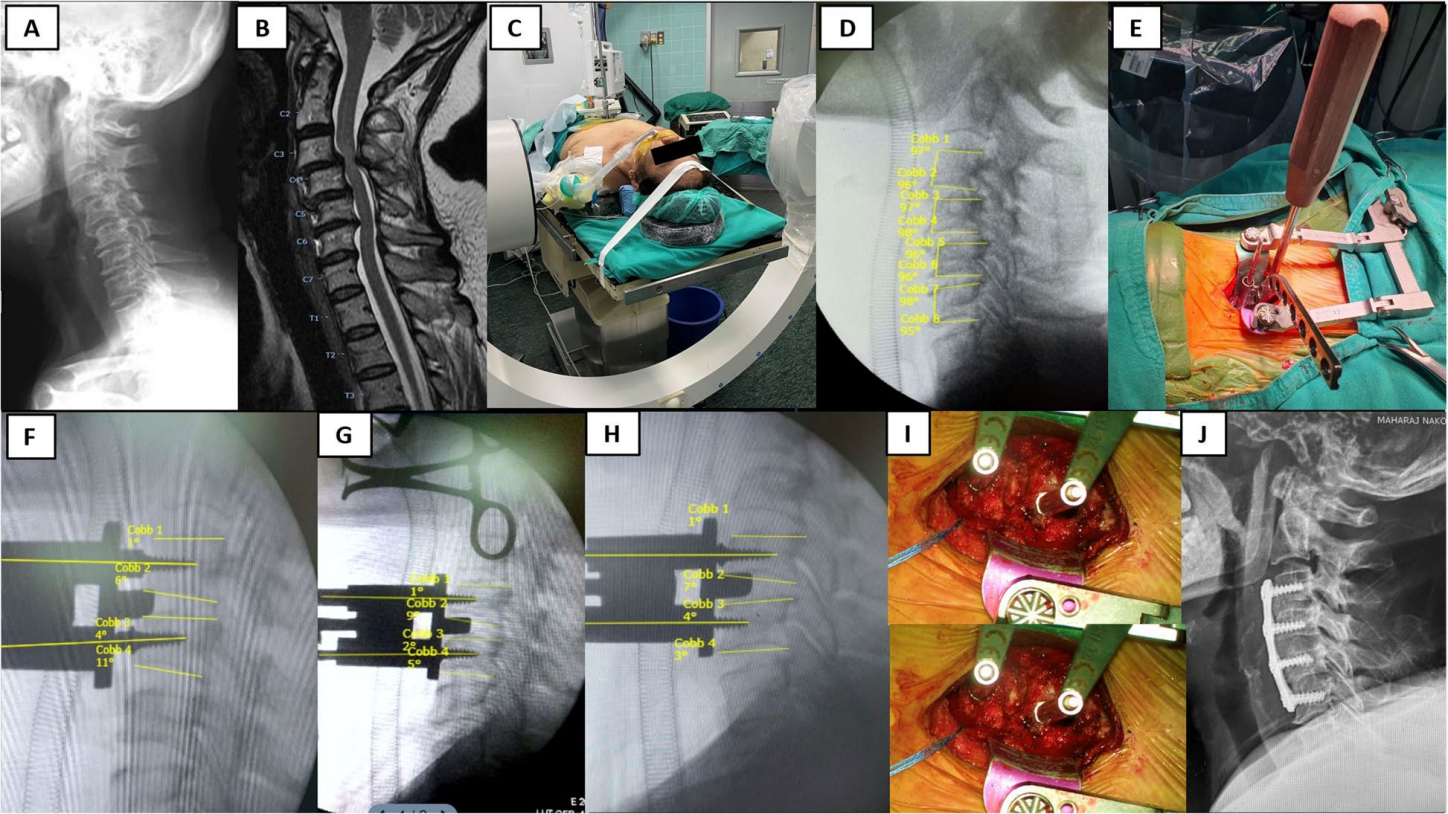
Patient 2 was performed Multilevel ACDF C3-4 C4-5 C5-6. Preoperative lateral X-ray (A), Sagittal MRI (B), Patient positioning (C), Intraoperative measurement of C3-C4-C5-C6 superior and inferior endplate angle (D), Caspar pin placement using the aiming device (E), C3-4 Caspar pin placement (F), C4-5 Caspar pin placement (G), C5-6 Caspar pin placement (H), Caspar cervical distractor system creating the working spaces (I), Postoperative lateral X-ray (J)
